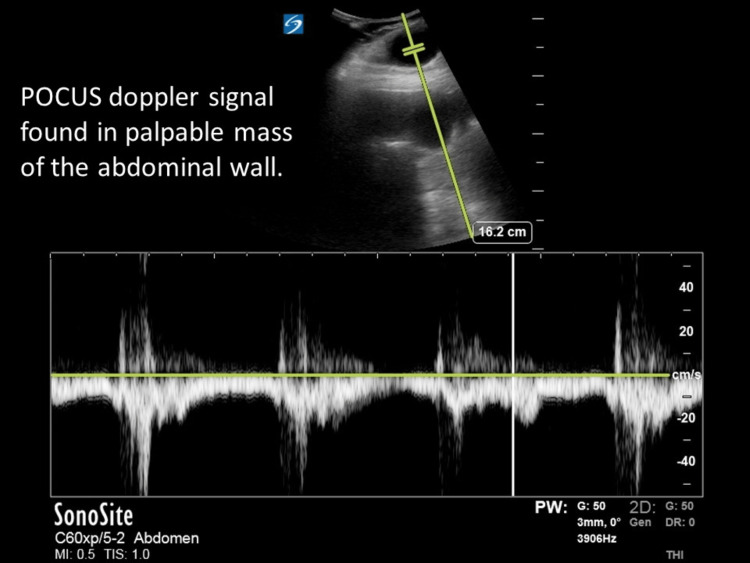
POCUS Doppler showing pulsatile flow in abdominal mass. POCUS: point-of-care ultrasound
